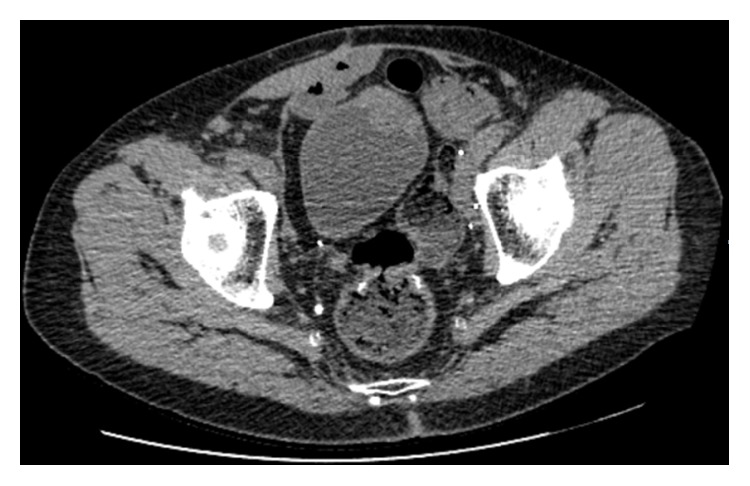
Abdominal CT-scan: lesion of the anterior wall of the neobladder.
